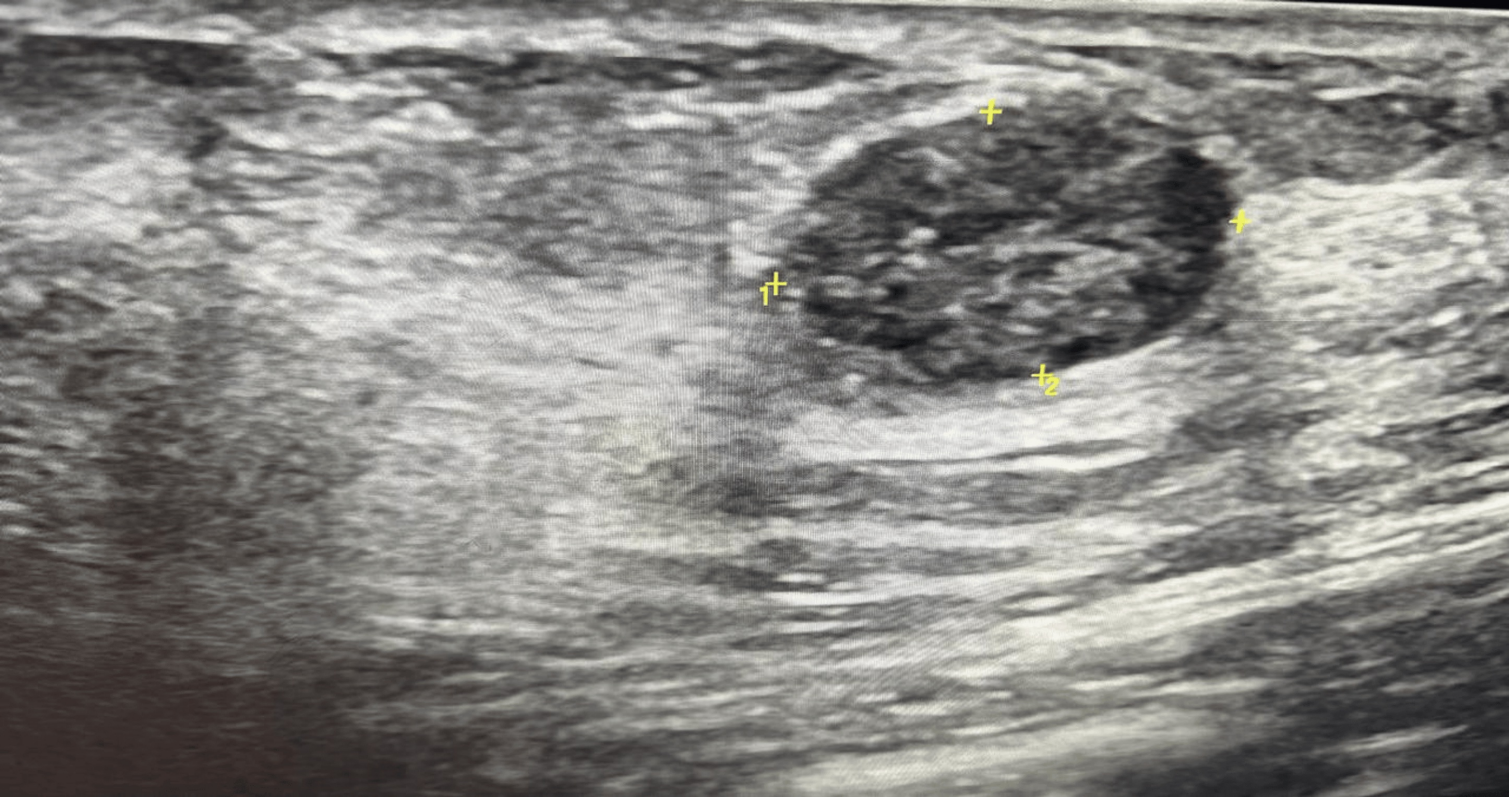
Ultrasonography findings of left breast: hypoechoic lesions with some calcification
